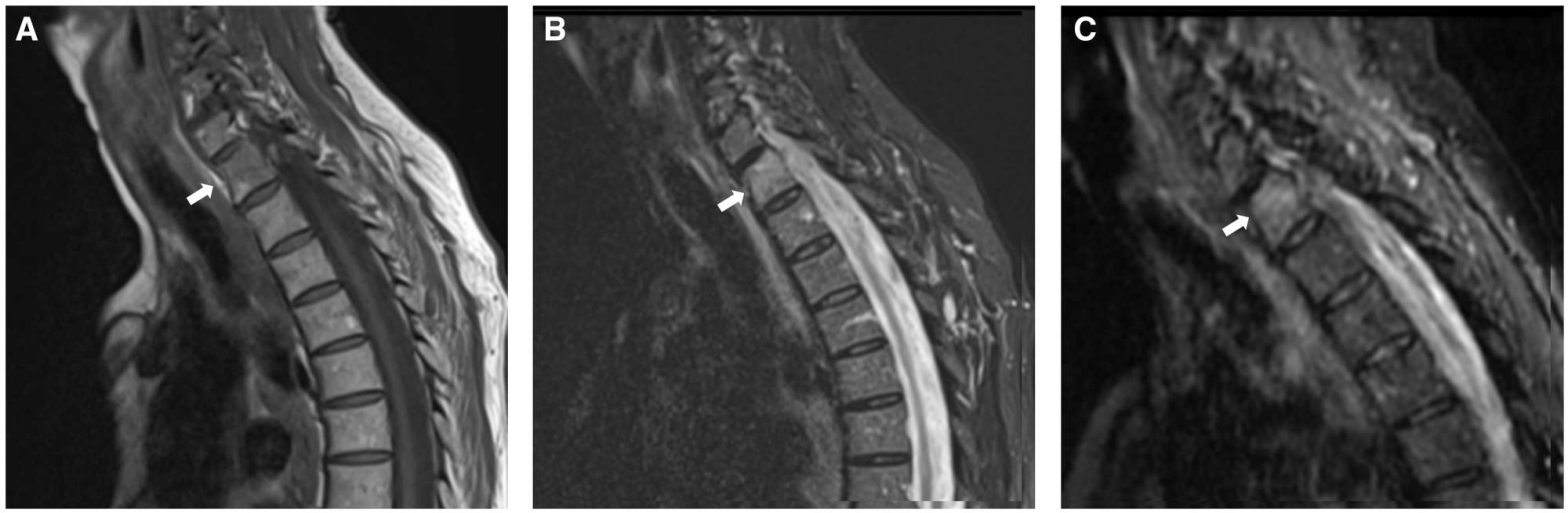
T1 vertebral body abnormal lesion (white arrow) in a 67-year-old female being investigated for mediastinal lymphadenopathy. (A, B) Sagittal MRI sequences demonstrate reduced T1w (A) signal, higher than the adjacent disc signal, and subtle increase in STIR signal (B) within the lesion. (C) Sagittal STIR MRI sequence performed twelve months later demonstrates stable signal characteristics (C).
